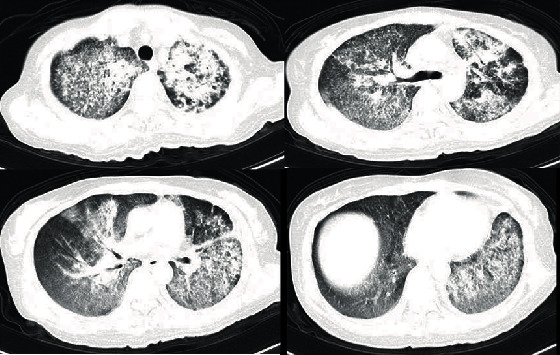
Characteristics of chest CT scan changes in patient.
